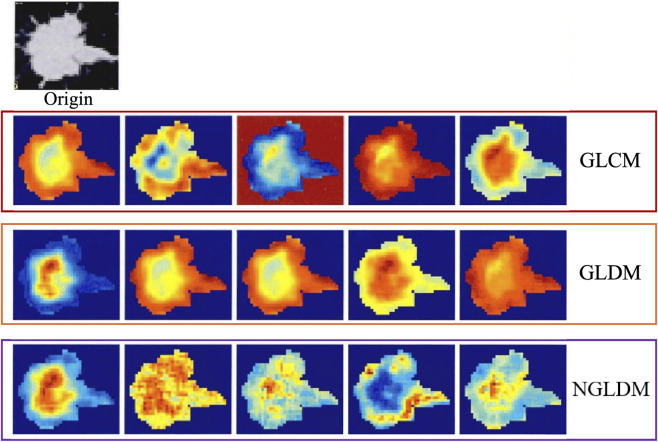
Original image and extracted CT grayscale features.
